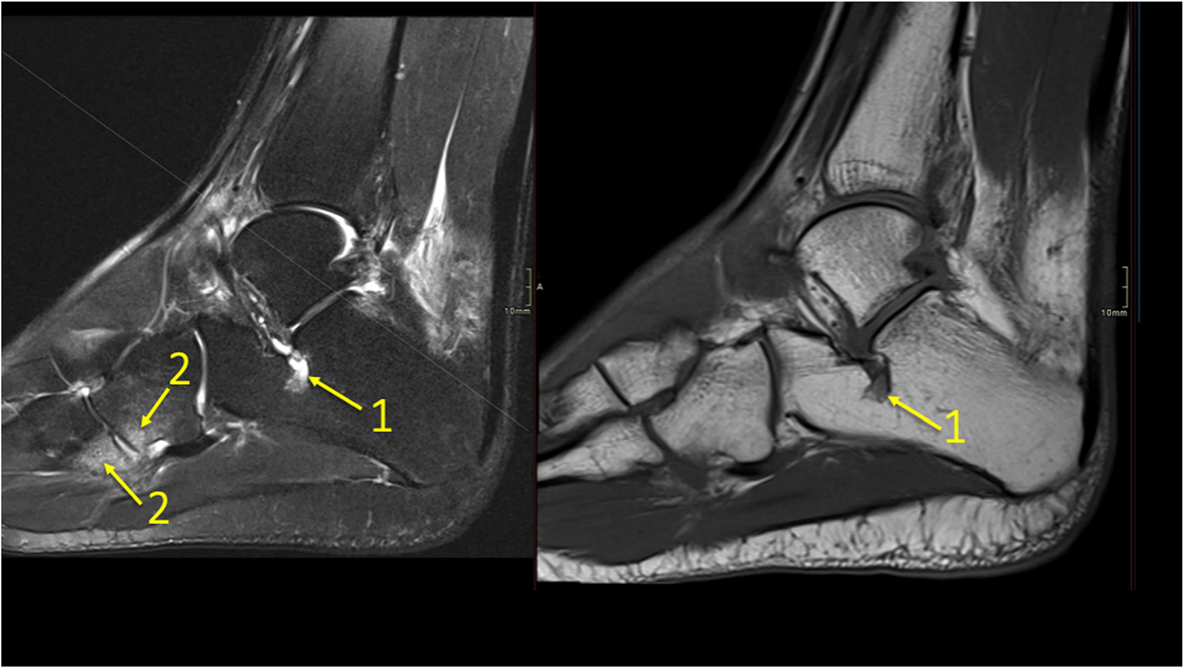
A 30-year old patient after ankle joint sprain. Radiographs revealed a Lisfranc fracture-dislocation. (1) MRI showed a vascular remnant, a pitfall, in the calcaneus. (2) BME was seen at the level of the Lisfranc joint
